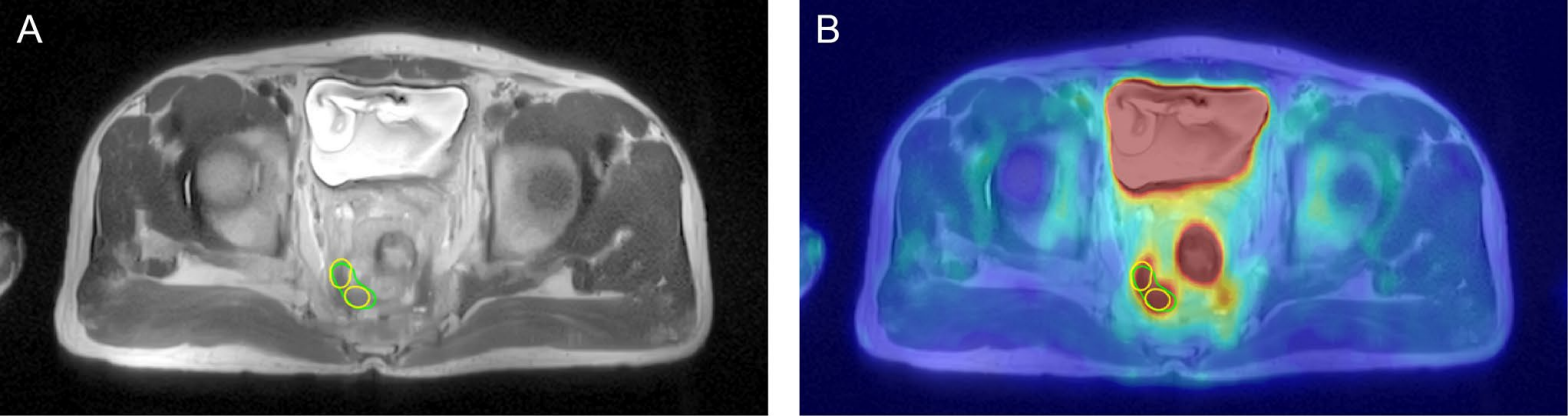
GTVn delineated for a 52 years old male on MRI (A) and PET/MRI (B). MRI image (A) delineated two smaller target volumes (yellow line) due to blurred boundaries, and PET/MRI image (B) showed complete GTVn (green line)
